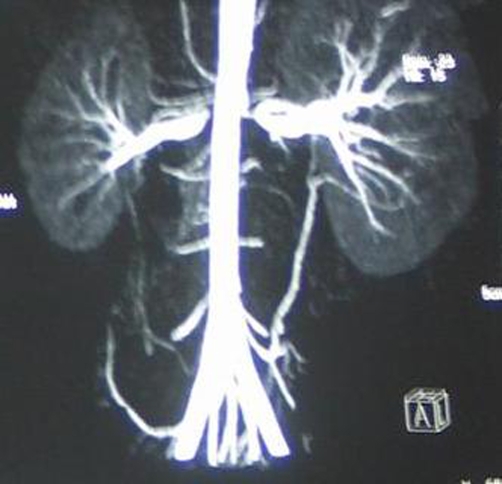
Computed tomography angiography of the renal arteries. Severe stenosis is visible in the proximal portions of the renal arteries bilaterally.
